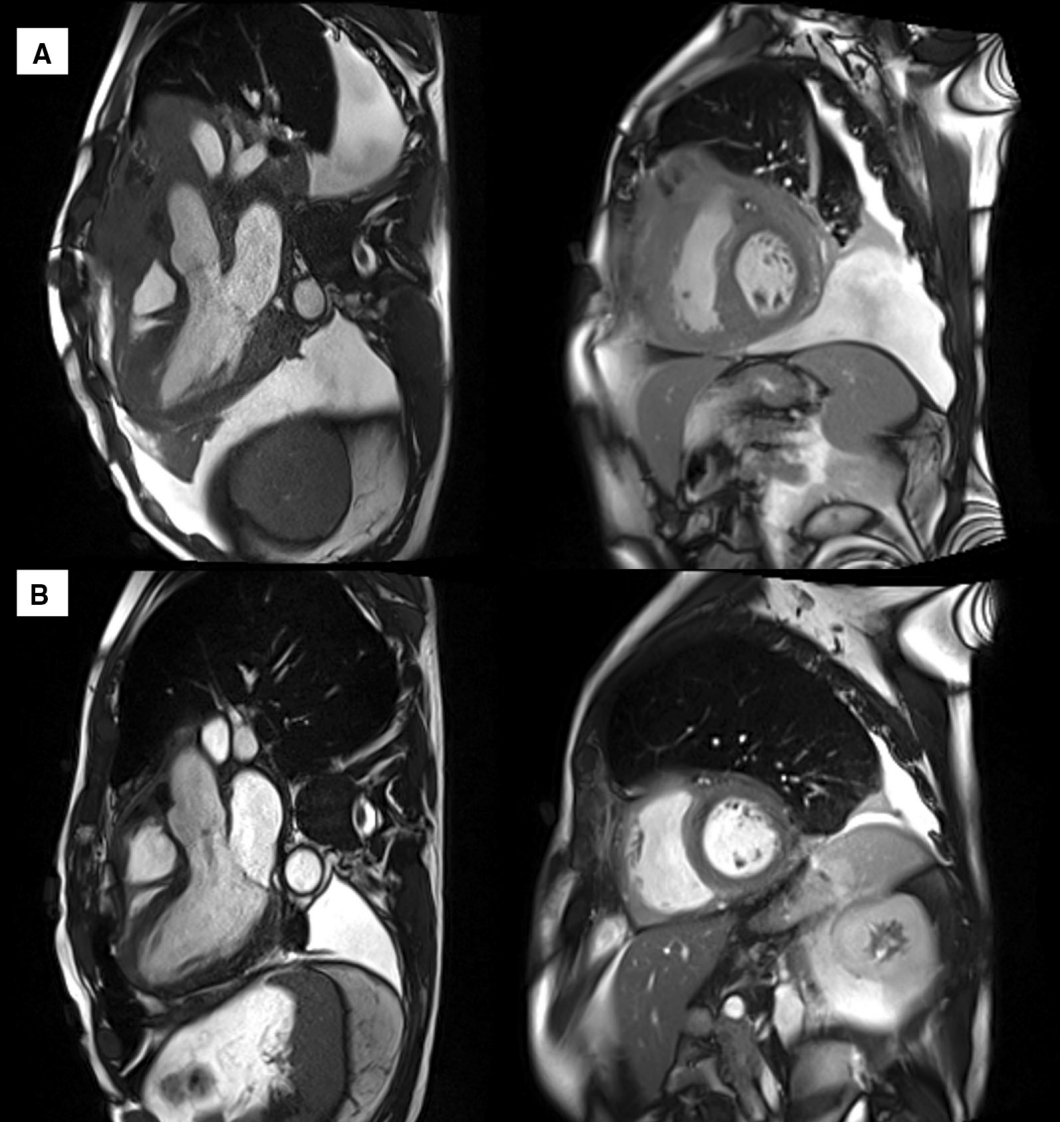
Cardiac magnetic resonance. A 3-chamber view and mid-ventricular short-axis view before chemotherapeutic treatment. Note the massive invasion of the heart by the mediastinal mass, whose cleavage borders are not evaluable; the myocardium appears thickened. Diffuse edema in T2-weighted sequences was detected, with a similar diffuse LGE pattern. B After the first regimen of chemotherapy, no more myocardial edema and late gadolinium enhancement were noticed, with near-normalization of left ventricle thickness
